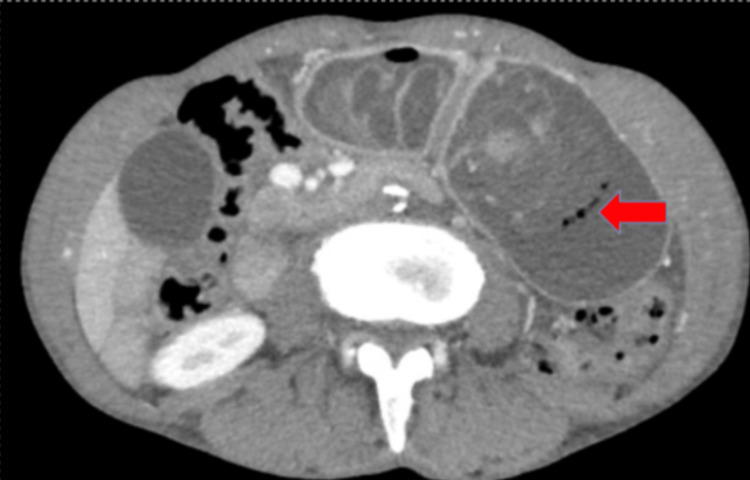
Axial CECT image shows invaginated jejunal loops into the stomach with pneumatosis within the wall suggesting bowel ischemia (red arrow). CECT: Contrast-enhanced computed tomography.
